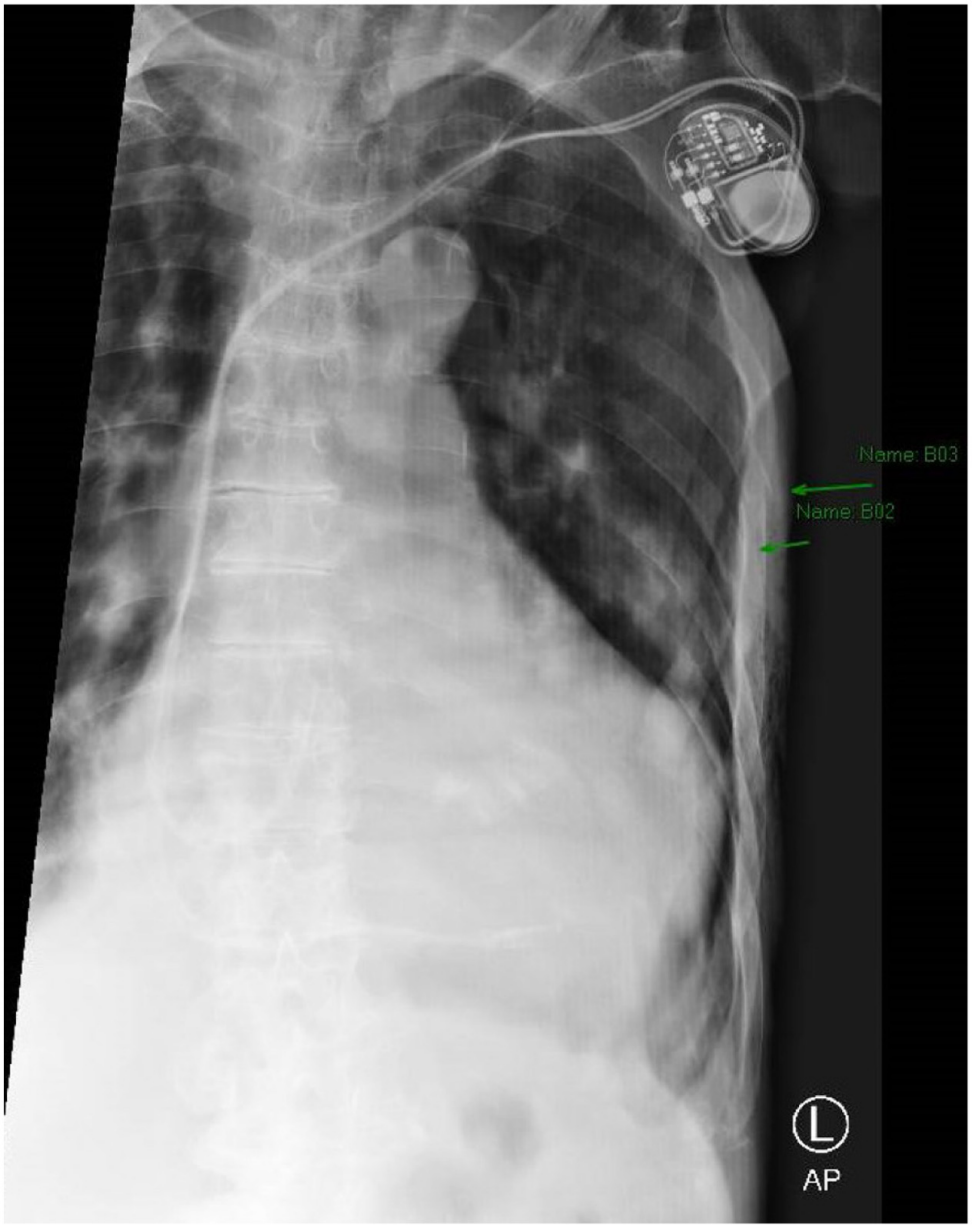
Magnified view of the chest radiograph demonstrating fractures of ribs five and six (arrows).
